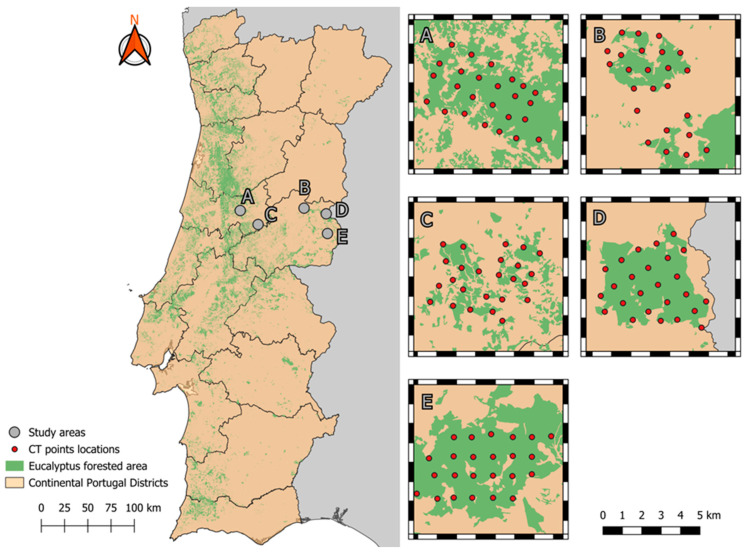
Selected study areas (grey points: A—Góis; B—Fundão; C—Pampilhosa; D—Penamacor; E—Penha Garcia) dominated by Eucalyptus plantations (green area) in Continental Portugal. The red dots represent the camera traps (CT) deployed in the study areas during one of the sampling periods.
